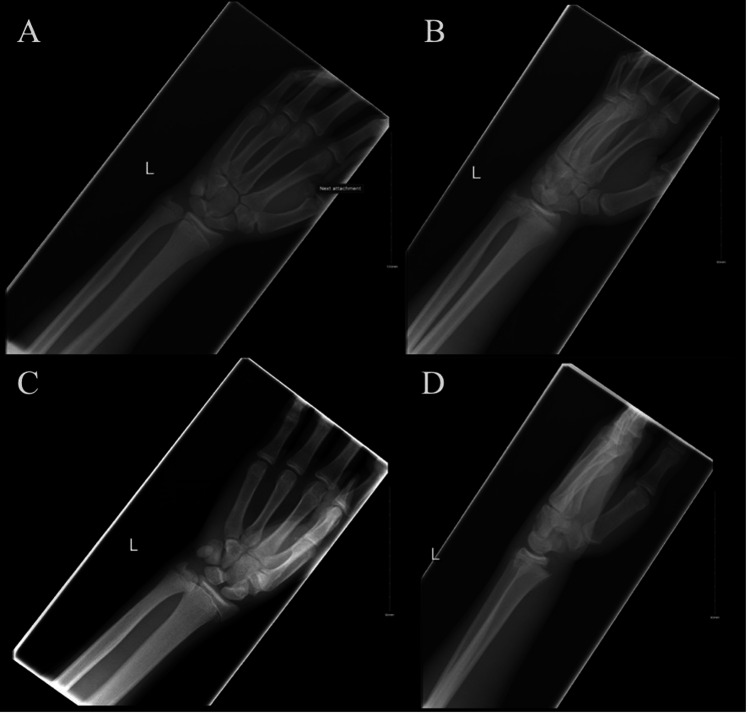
Left wrist radiographs pre-reduction Postero-anterior (A), oblique (B and C), and lateral (D) radiographs of the left wrist pre-reduction demonstrating left Salter-Harris II distal radius fracture with dorsal angulation.
